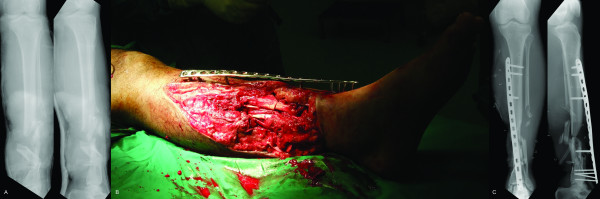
a - Comminuted Gustillo-Anderson IIIB open diaphyseal fractures of the right tibia and fibula. b - Exchange external fixation performed with LCP contoured to facilitate soft tissue coverage. c - Postoperative radiograph of LCP external fixation.
